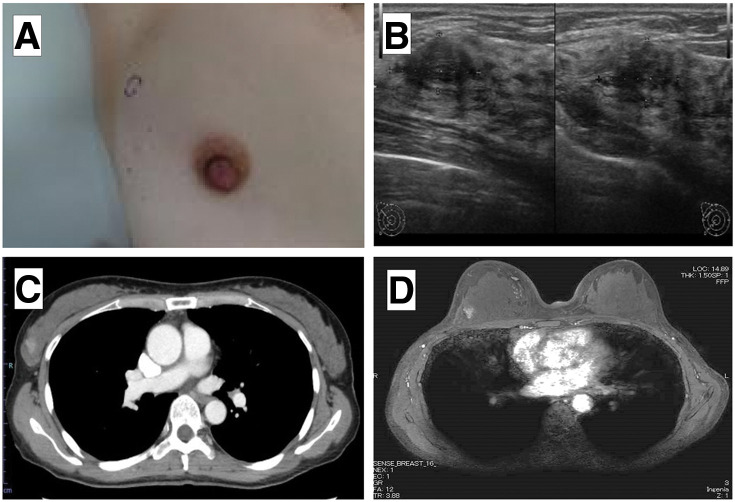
Clinical and imaging findings. (A) Physical findings of the right breast at presentation. (B) Breast ultrasonography showing a 1.3-cm mass in the upper outer quadrant of the right breast. (C) CT showing no evidence of distant metastasis. (D) Breast MRI showing no apparent intraductal extension.
